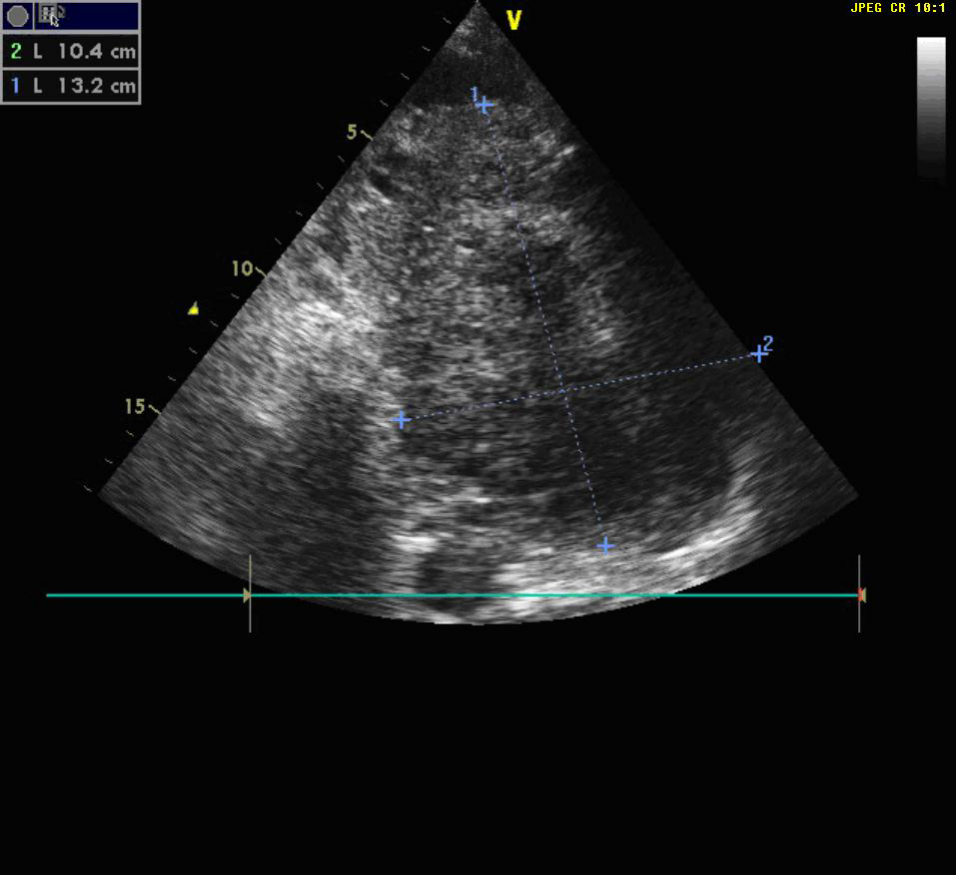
Sonography demonstrating a giant mass in the left pleural space penetrating the left pulmonary veins.
